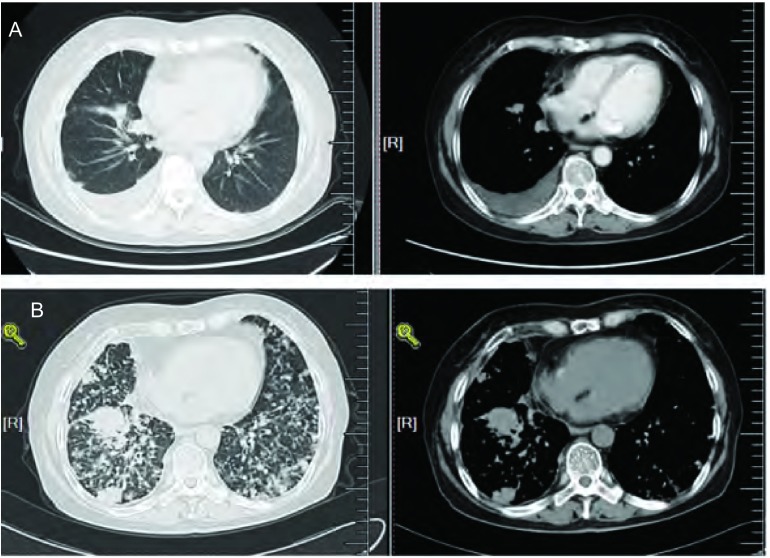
患者治疗过程中的CT结果。A：2009年4月患者入我院时的胸部CT示右肺癌和胸腔积液；B：2012年2月患者胸部CT示右肺癌伴广泛转移。 CT scan of the chest during the therapy. A: CT scan of the chest showing a soft tissue mass and pleural effussion at right lung; B: CT scan of the chest showing right lung caner and extensive metastasis.
